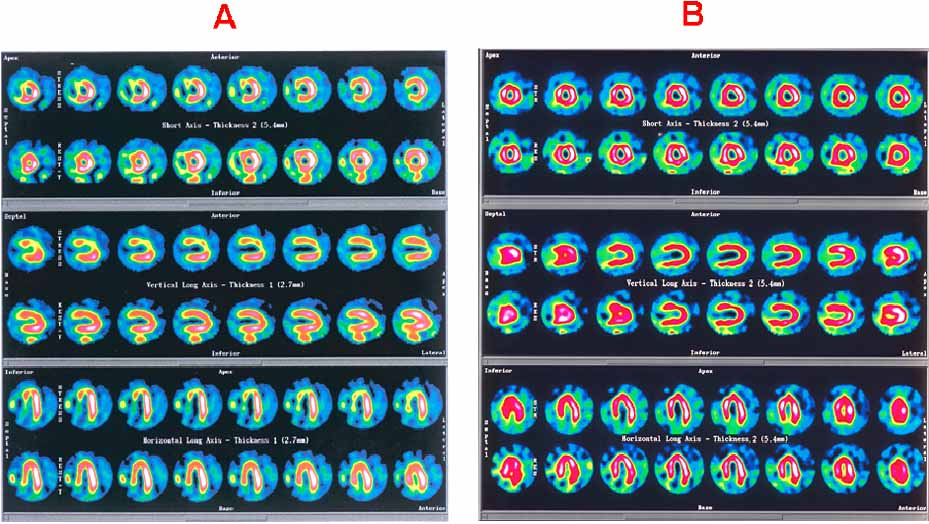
Myocardial perfusion SPECT imaging of a 61-years old patient with one-vessel disease (LAD 95%) before (A) and 6 months after (B) LAD PCI, demonstrated significant improvement in the apex, anterior wall and septum.
